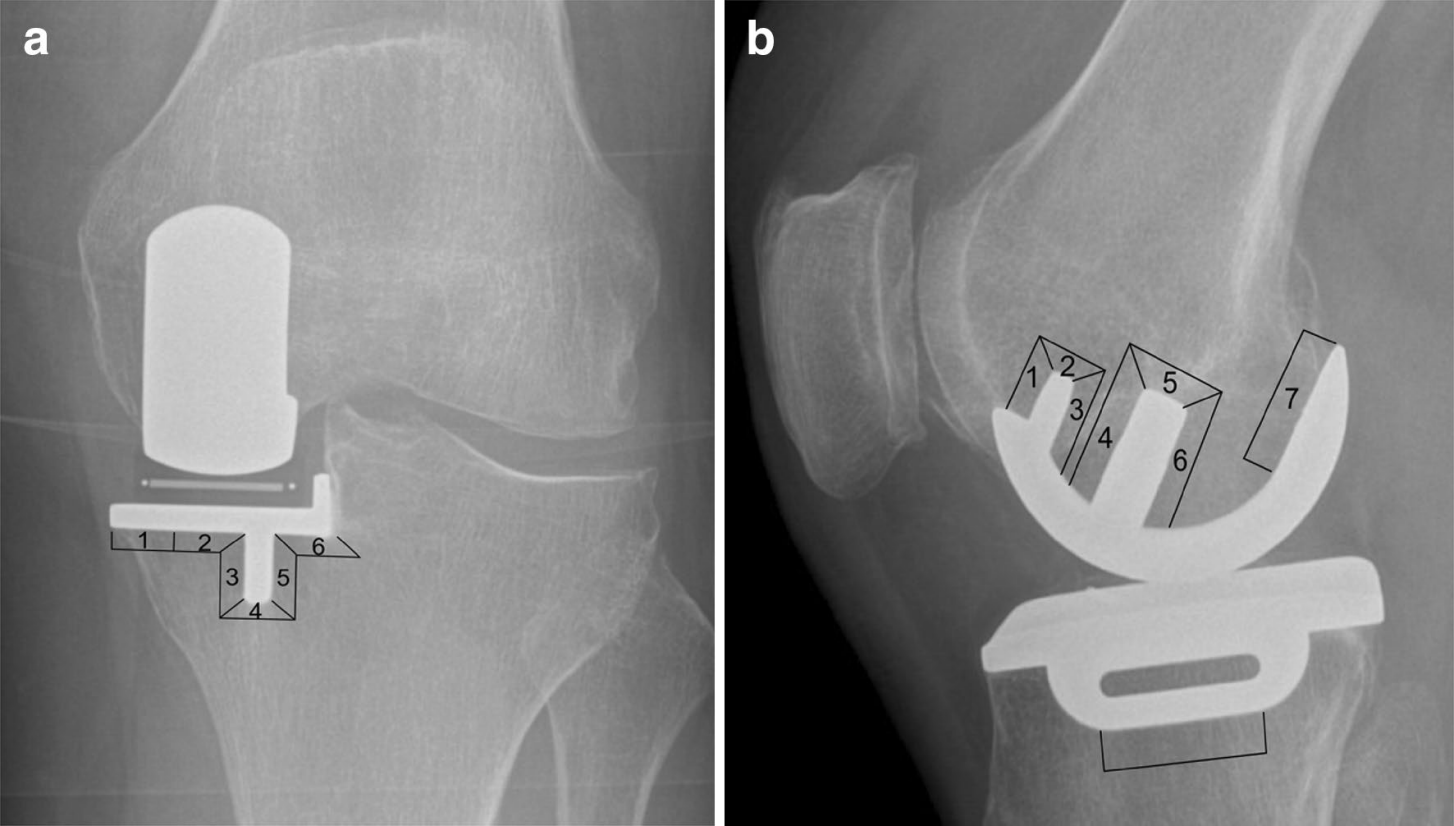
Zones assessed for radiolucencies on radiographs. a Anteroposterior radiograph, b lateral radiograph
